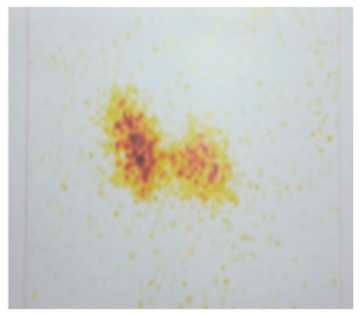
Thyroid scintigraphy with hypo-uptake in the left thyroid lobe, slight hypo-uptake in the right lobe, without suspect scintigraphy nodules.
